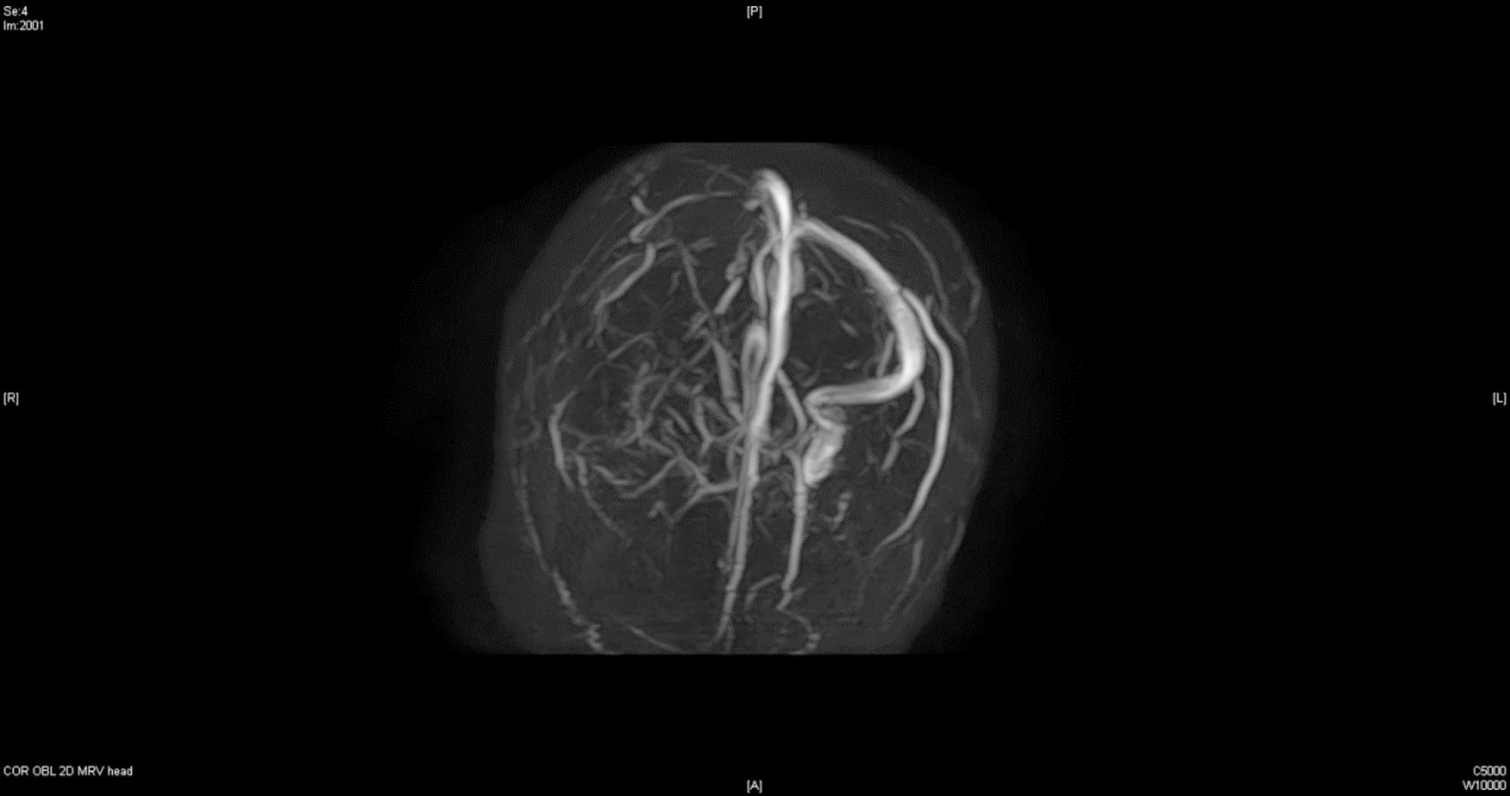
Magnetic resonance imaging (MRI) venogram brain without contrast, day 1.
